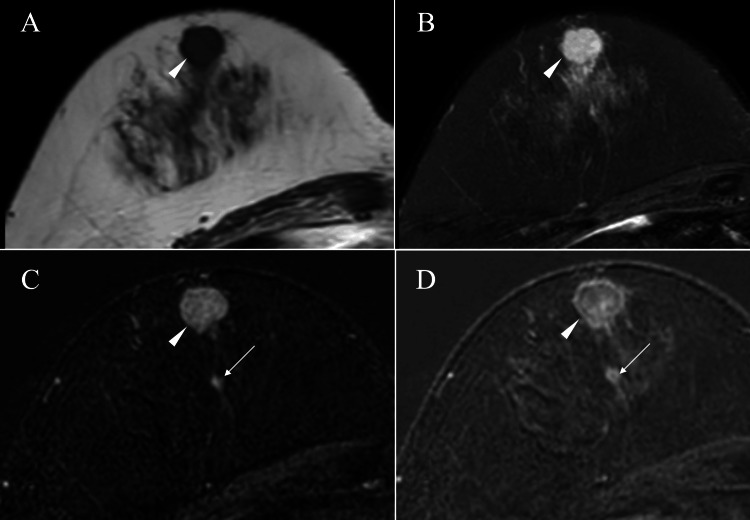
Magnetic resonance imaging (MRI) findings MRI of the main tumor showed low signals on T1-weighted images (A, arrowhead), high signals on fat-suppressed T2-weighted images (B, arrowhead), and a washout pattern on dynamic studies (C and D, arrowhead). MRI further showed a daughter nodule (C and D, arrow) in the peripheral areas of the main tumor.
